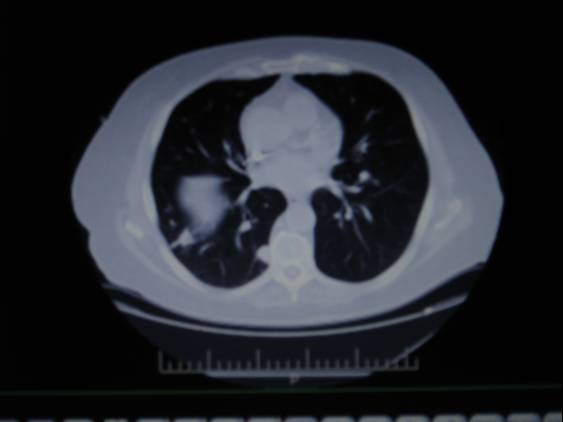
Chest CT scan. Nodule involving the right lower lobe, medially, measuring approximately 1.2 × 1 cm, seen adjacent to the thoracic spine
